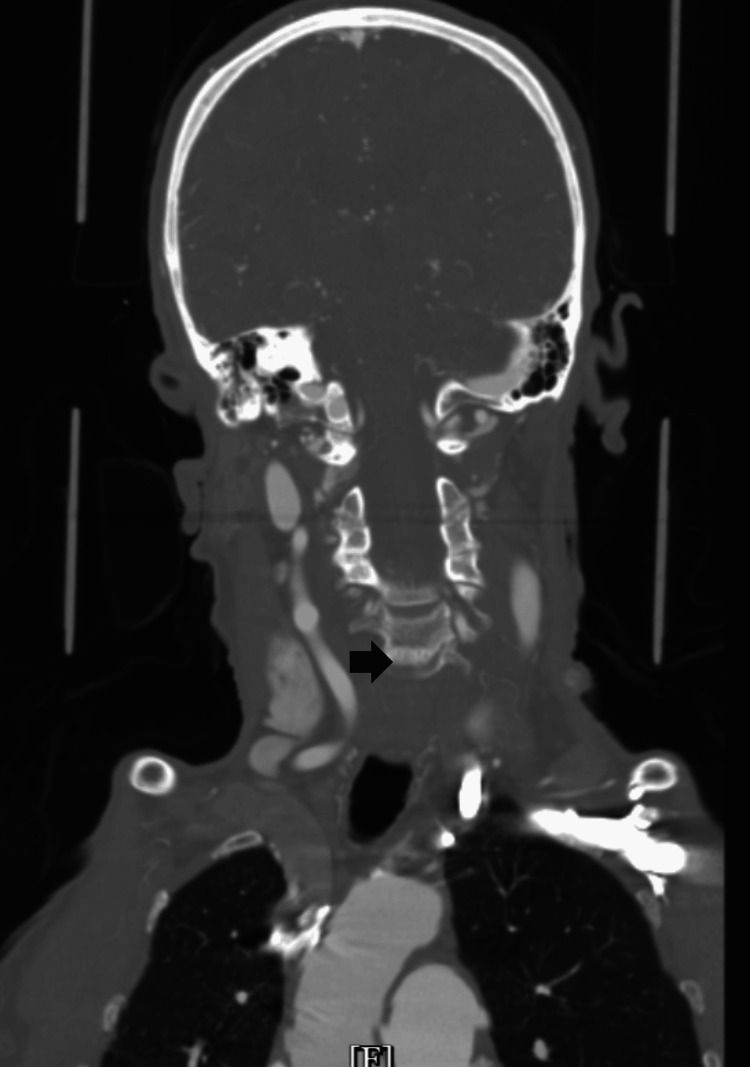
CTA showing occluded common and internal carotid on the left side (indicated by the black arrow, with the right side showing a patent carotid vessel). CTA: computed tomography angiography
